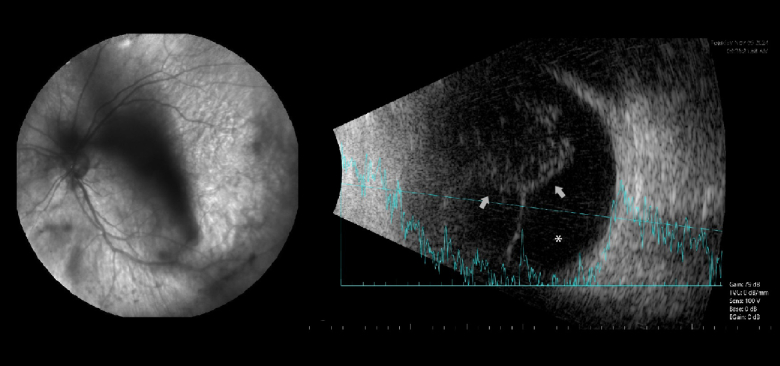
Left: The infrared image of the fundus reveals the shadow of the partially detached vitreous body over the macula. Right: B-scan ultrasonography (gain: 79 dB) demonstrates a detached vitreous body that retains attachments to the peripheral retina (incomplete PVD). The echogenic opacities are mainly confined to the thickened, irregular peripheral vitreous cortex (arrows). Note the echo-free space between the detached vitreous body and the retina (asterisk).
